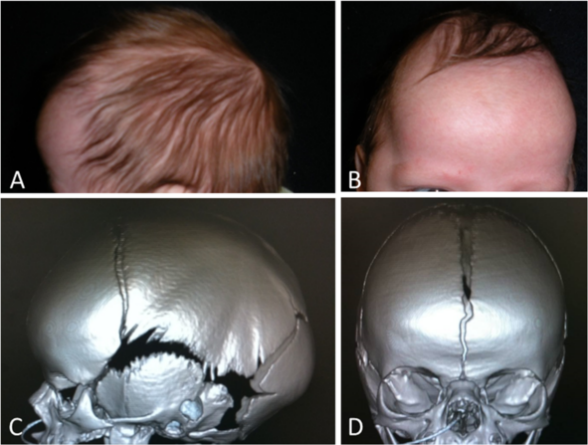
Scaphocephaly from sagittal craniosynostosis. Phenotypic photographs (A, B) and computed tomography imaging (C, D) showing preoperative scaphocephaly from sagittal craniosynostosis.
